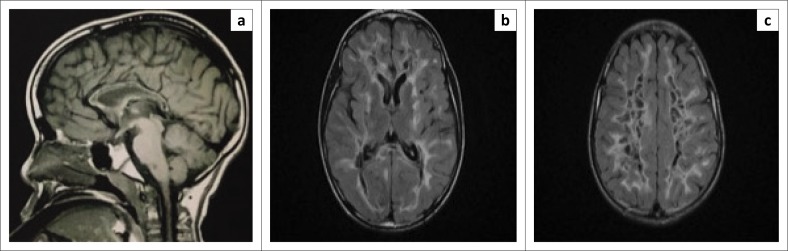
Magnetic resonance imaging after 2 years: (a) sagittal T1 weighted imaging (T1WI) demonstrates involvement of the inner rim of the corpus callosum. (b and c) Axial fluid attenuation inversion recovery (FLAIR) images with further progressive signal abnormalities and cystic degeneration of the white matter.
